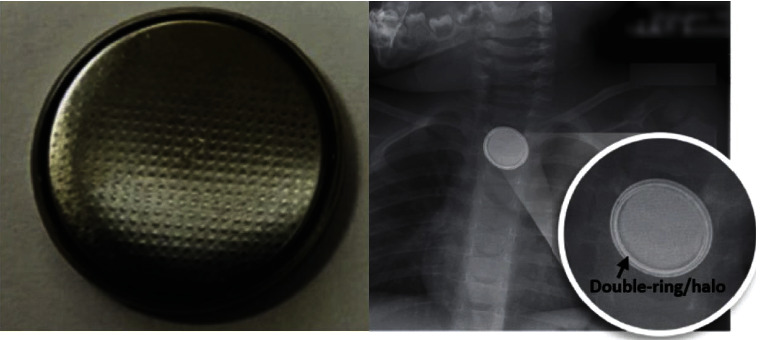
Button batteries are metallic, disc-shaped objects (left). They may be recognized on radiographs by identifying the characteristic “double-ring” or “halo” sign (right), reproduced with permission of KR Jatana.
